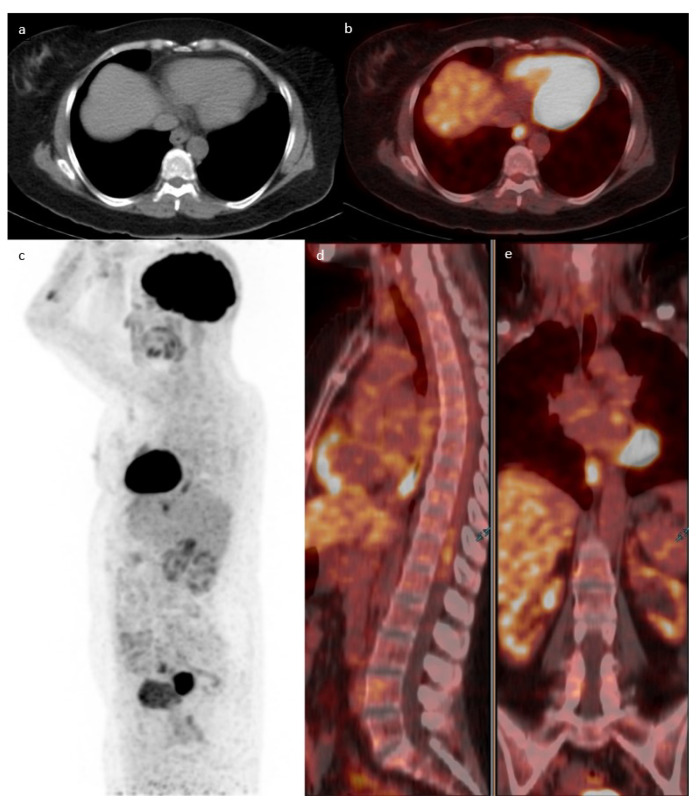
Case of a 70-year-old female known to have uterine cervical cancer. Axial CT (a) fused with PET (b) showing a focus of uptake in the distal esophagus with an SUVmax of 5.6. MIP (c)-fused sagittal (d) and axial PET/CT (e) showing increased linear uptake within the distal aspect of the esophagus. The patient underwent gastroscopy, which revealed inflammatory processes in keeping with esophagitis and no evidence of malignancy.
